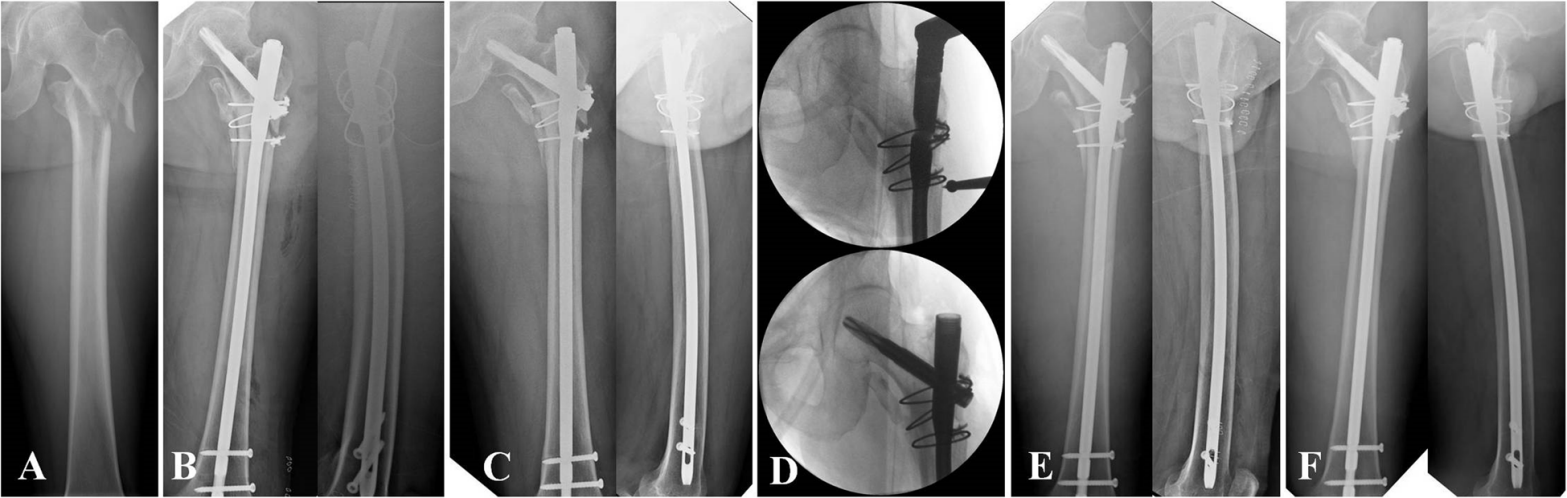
a A 44-year-old male patient. b Postoperative radiographs. c Nonunion and implant failure at 13 months after surgery. d Revision surgery using closed procedure without bone graft. e Postoperative radiographs. f Bony union at 7 months after revision surgery
